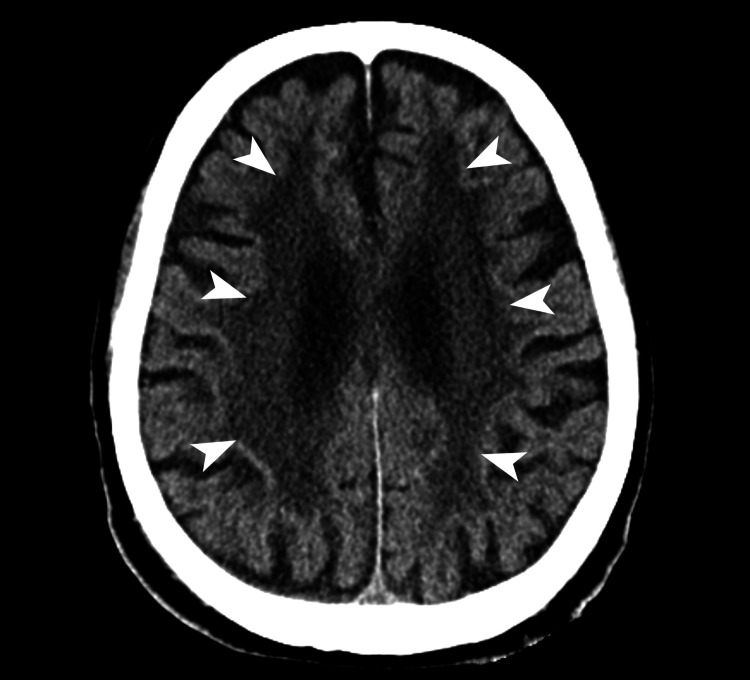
Non-contrast-enhanced head computed tomography performed in late October 2021 Atrophy of the cerebrum and diffuse low-density areas in the white matter (arrowheads) were noted.
